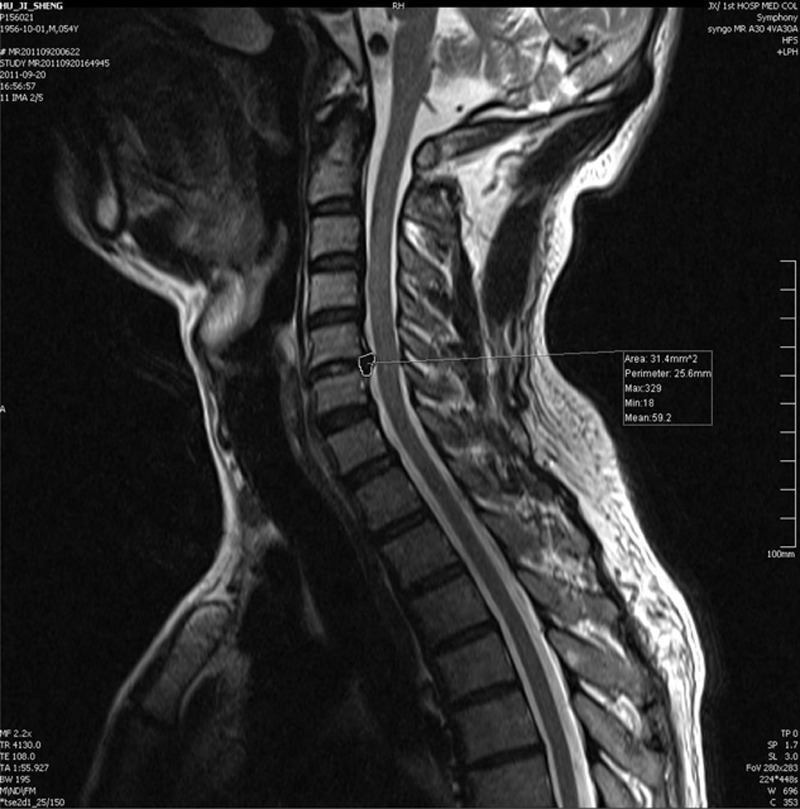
Protrusion area was measured and compared using magnetic resonance imaging and picture archiving and communication systems.
